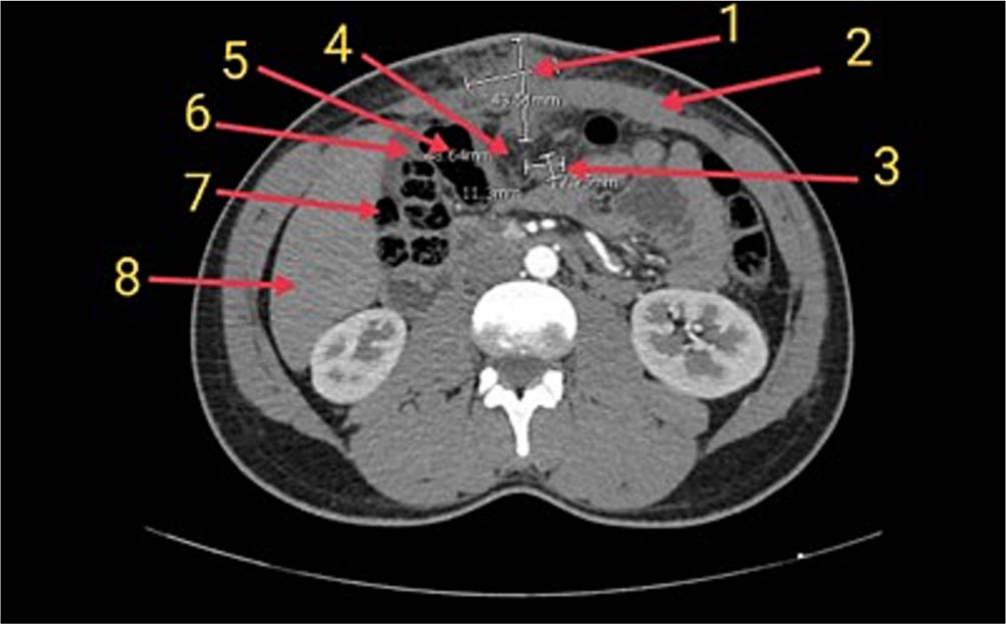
Axial CT image of the abdomen showing the inflammatory myofibroblastic tumor (1) in the supraumbilical anterior abdominal wall. The mass is closely associated with the rectus sheath (2), a presumed lymph node (3), and the greater omentum (4), which appears inseparable from the tumor. Adjacent bowel loops including the transverse colon (5), hepatic flexure (6), and ascending colon (7) are noted in close proximity. The liver is also partially visible (8) in the field.
